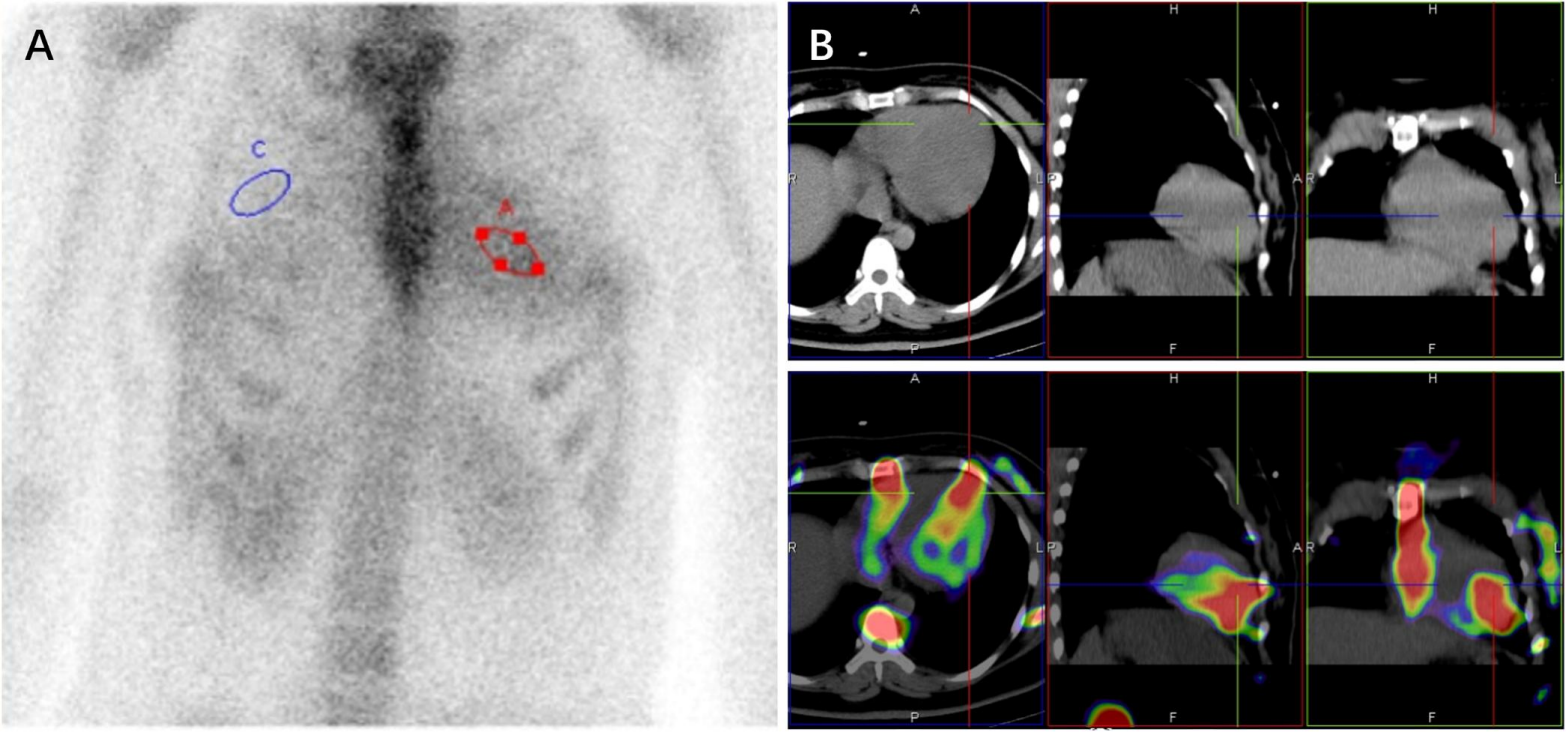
^99m^Tc-PYP scintigraphy (A) and SPECT/CT images (B) revealed a grade 3 myocardial uptake with heart (red circle) to contralateral lung (blue circle) ratio of 1.82 at 3hr (A).
